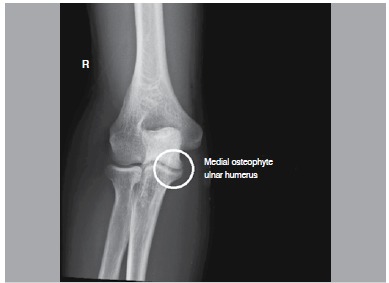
Radiographic alteration grade 1. Presence of medial osteophyte shown in the anteroposterior X-ray.
